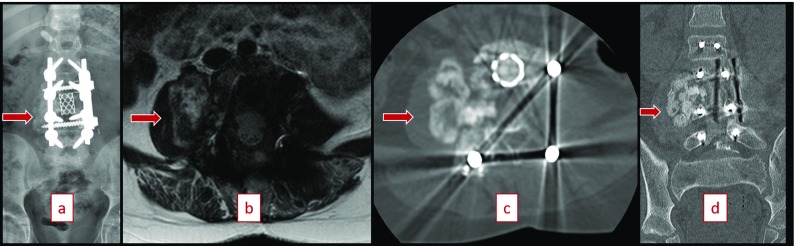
(a) PA of spine 9 months post initial resection with recurrence (November 2016). (b) T2 Axial MRI demonstrating recurrent osteosarcoma extending into right psoas muscle (December 2016). (c, d) CT scan cuts demonstrating recurrent osteosarcoma with previous retained implants (December 2016).
